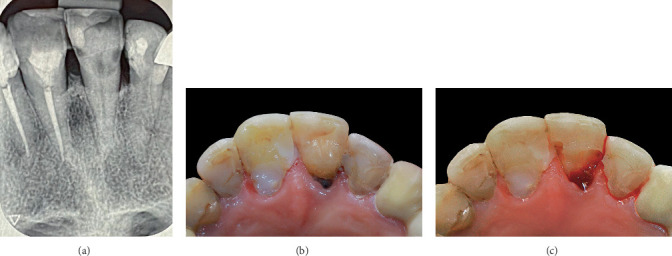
(a) Periapical radiograph of maxillary anterior teeth. (b) Initial image depicting the palatal surface of the maxillary anterior teeth. (c) Tooth 9 after caries removal.
